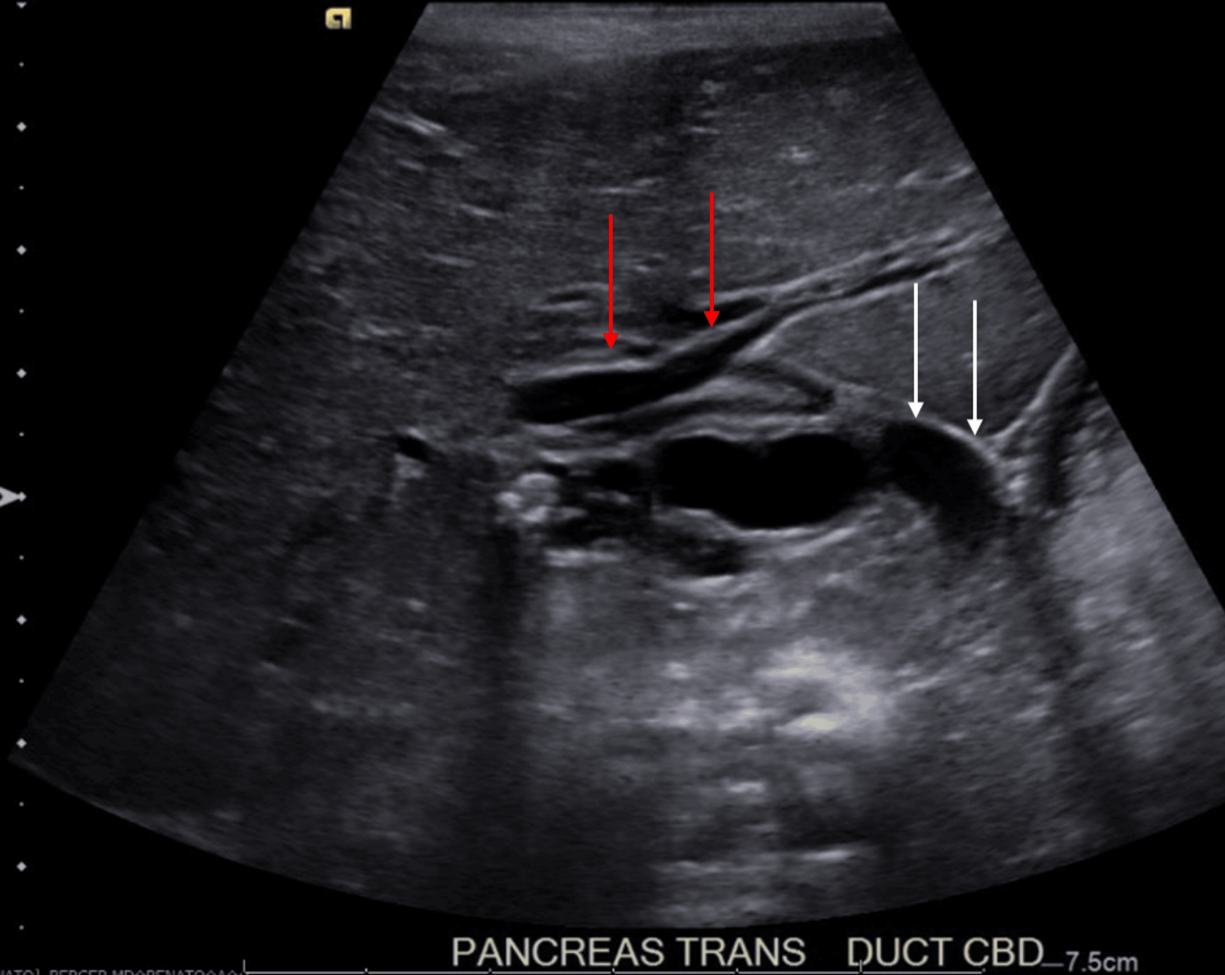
Initial abdominal ultrasound Abdominal ultrasound (pancreas transverse view) showing several stones within the gallbladder without gallbladder wall thickening, mild to moderate intra- and extrahepatic biliary ductal dilatation with the common bile duct measuring 9 mm and an ovoid lesion measuring 1.5×0.6 cm in the distal common bile duct. Red arrows point to intrahepatic biliary ductal dilatation. White arrows point to dilated gallbladder and extrahepatic biliary ductal dilatation.
